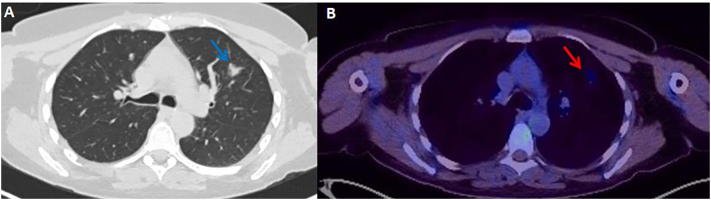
PET-CT demonstrates a 16 mm spiculate solid mass in the left upper lobe (panel A, left hand side, blue) on CT reconstruction. The PET component (panel B, right hand side, red arrow) shows the same mass taking up low levels of FDG tracer.
